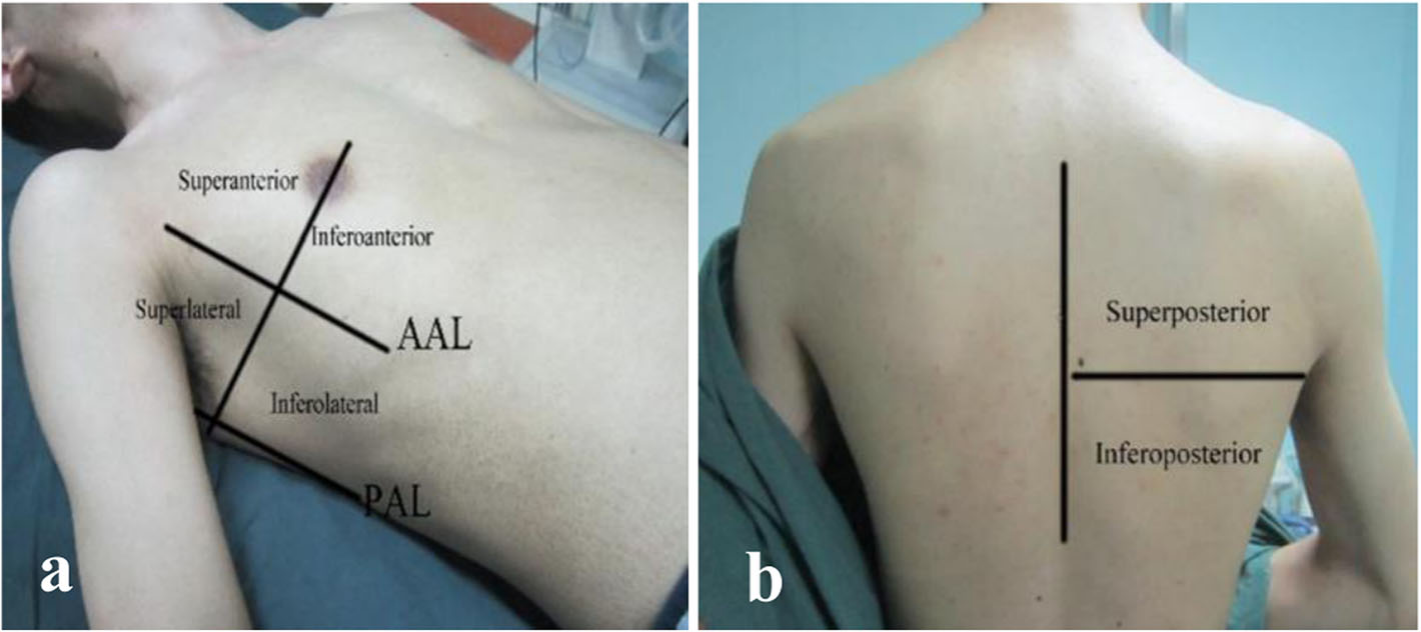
Hemithorax partition during lung ultrasound examination. a, b Each hemithorax was divided into 6 quadrants by anterior and posterior axillary lines. Abbreviations: AAL, anterior axillary line; PAL, posterior axillary line. Xie et al. 2020 [2]
